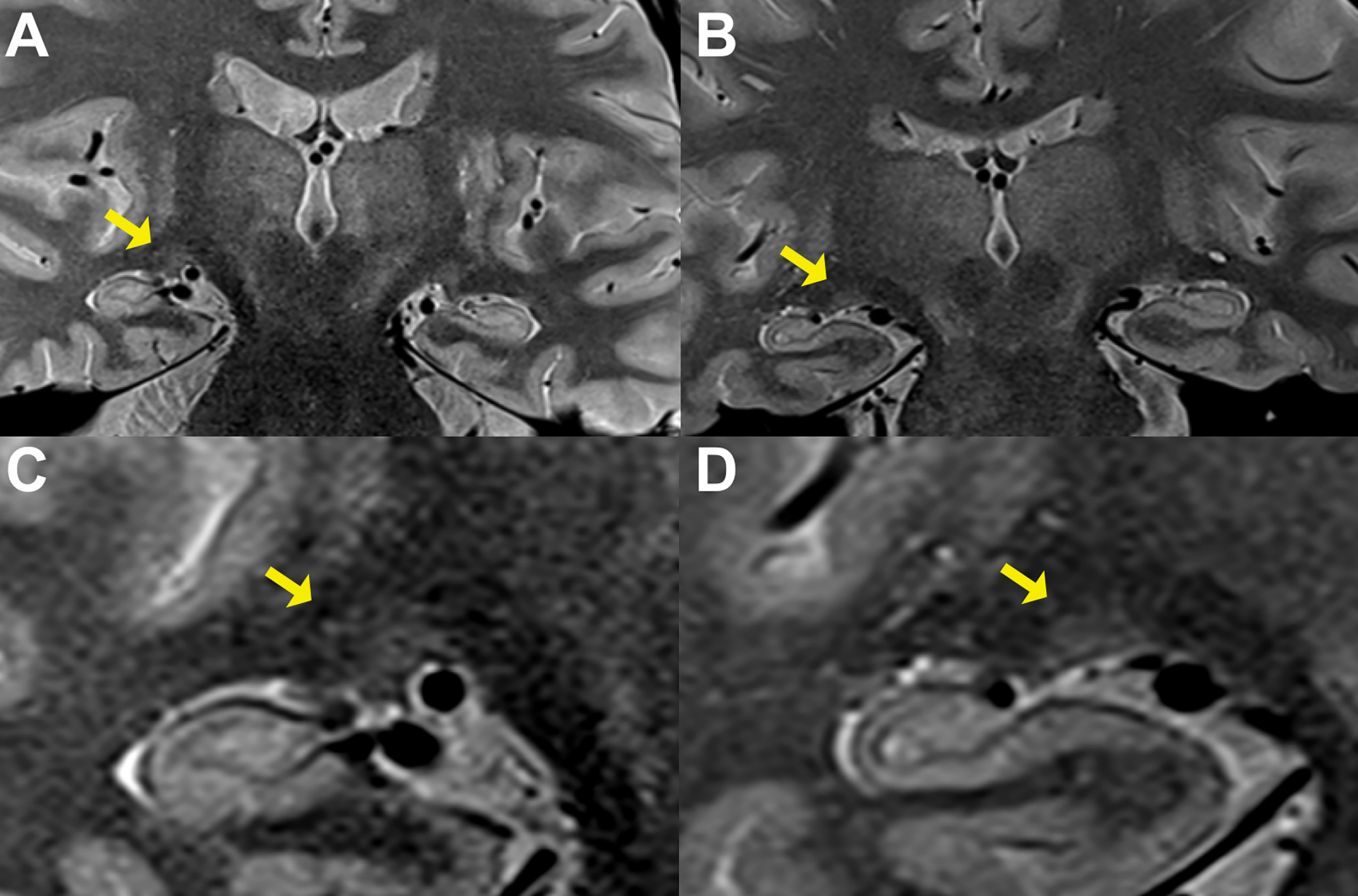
Magnetic resonance imaging scans showing the lateral geniculate nucleus (LGN). *A*, right LGN of a healthy individual (yellow arrow); *B*, right LGN of a glaucoma patient; *C*, zoomed-in image of the same LGN (yellow arrow) in image A; *D*, zoomed-in image of the same LGN (yellow arrow) in image B.
